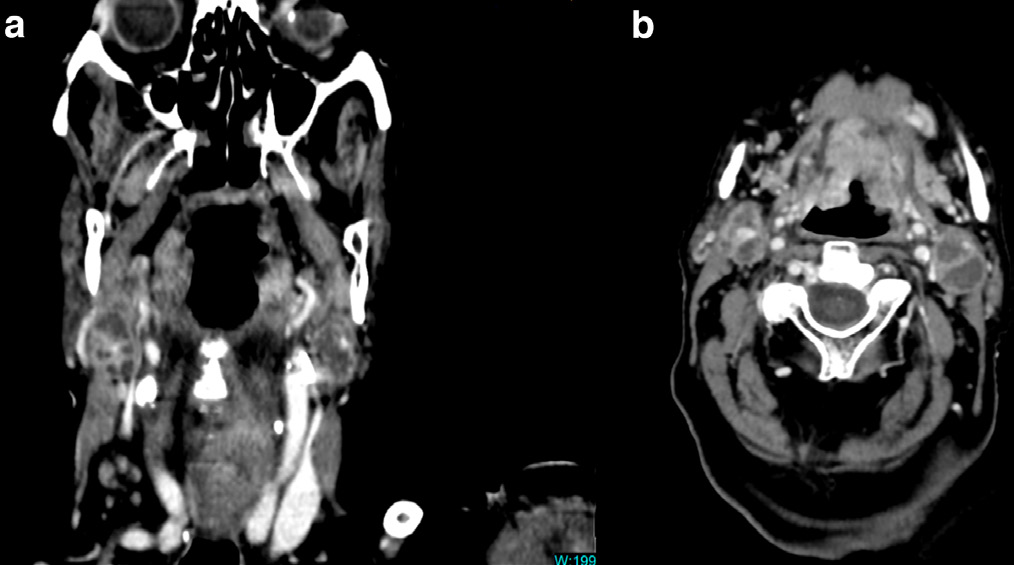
Level II A ill-defined bilateral necrotic lymphadenopathies in coronal (A) and transverse (B) venous phase CT images. Irregular capsular enhancement and obliteration of fat planes next to the sternocleidomastoid muscles suggest extranodal invasion.
